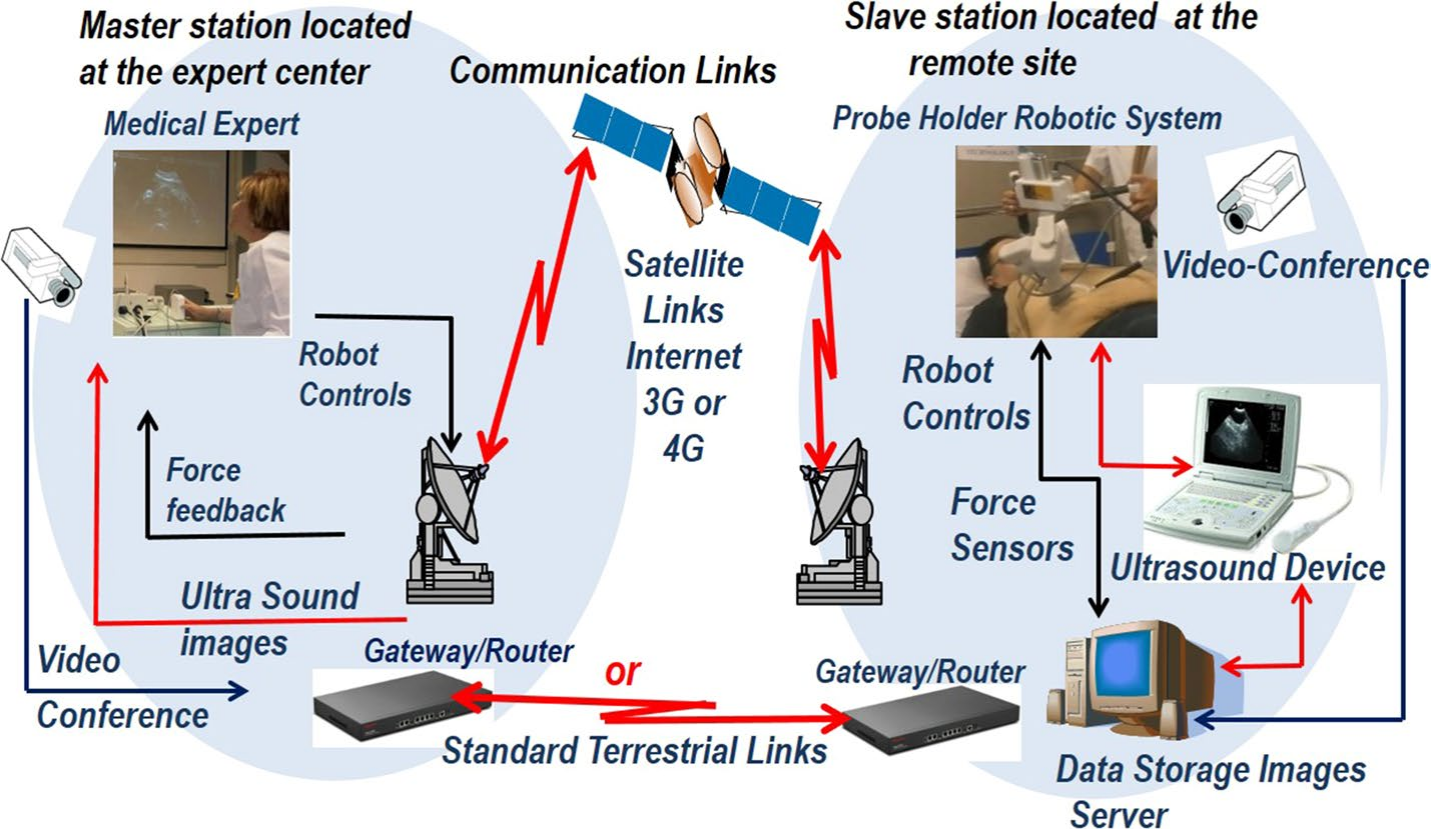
Robotized tele-ultrasound using the MELODY system [54]
